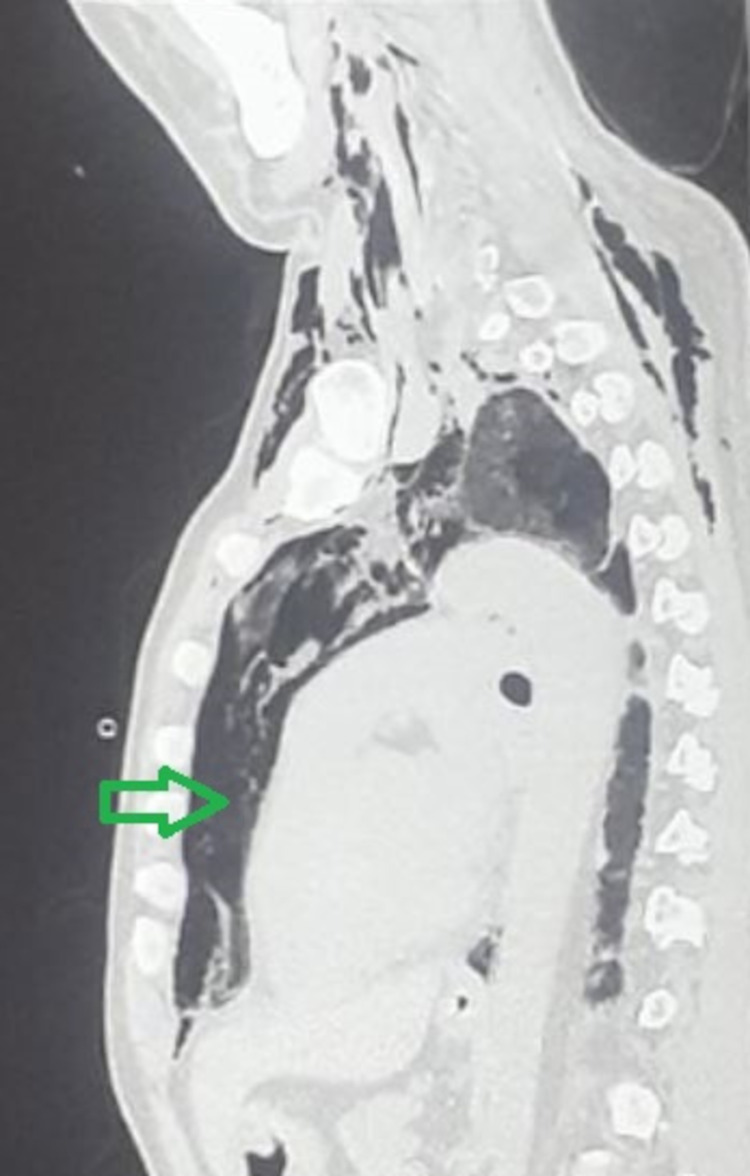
Left Parasagittal slice of CT image showing finding of pneumomediastinum (shown by a green arrow)
